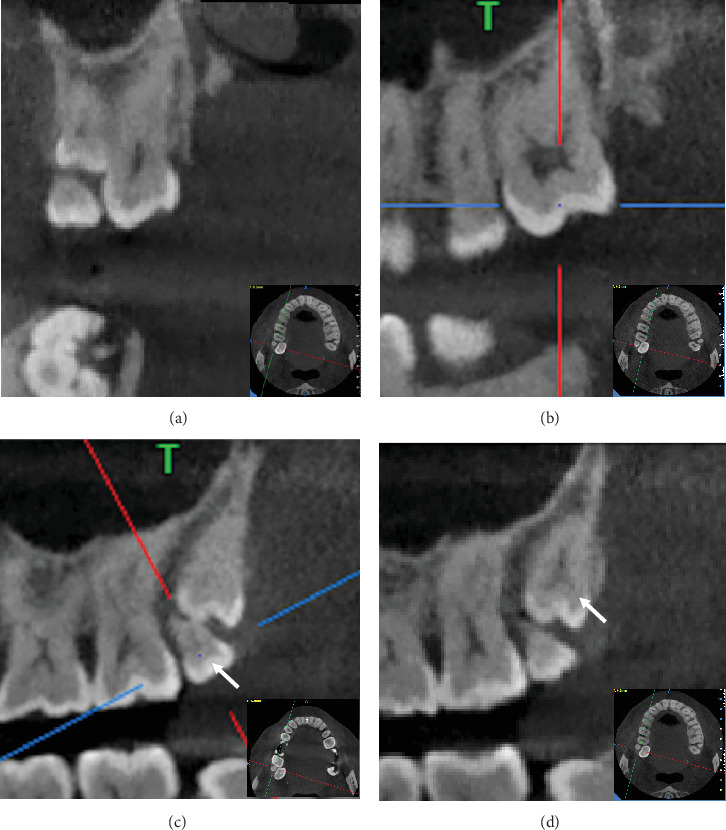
(a) Coronal view of the patient's CBCT scan showing the maxillary third, fourth, and fifth molars in the right maxillary quadrant. (b–d) Sagittal views of the maxillary (b) third, (c) fourth, and (d) fifth molars in the right maxillary quadrant. The white arrow points to the right maxillary fourth molar.
